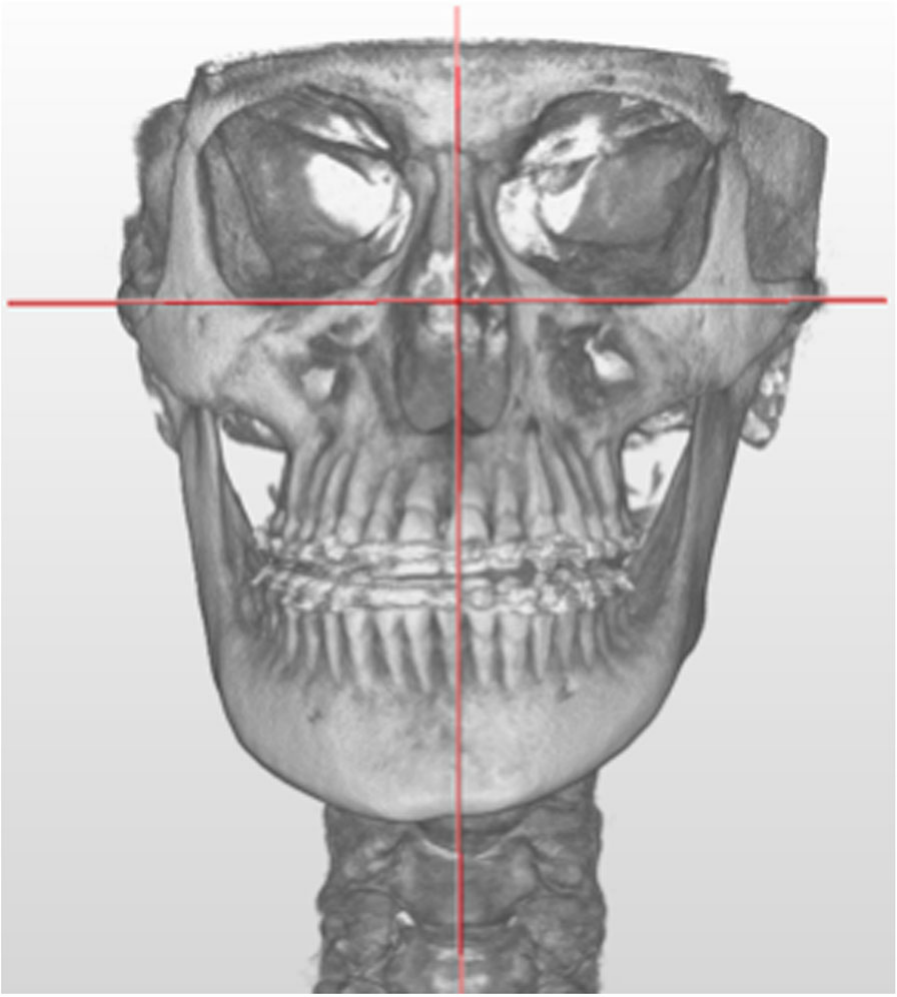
3D CT-reconstruction performed using CT data of PET/CT scan: specific software allows a computer-assisted three-dimensional surgical planning; patient with UCH
